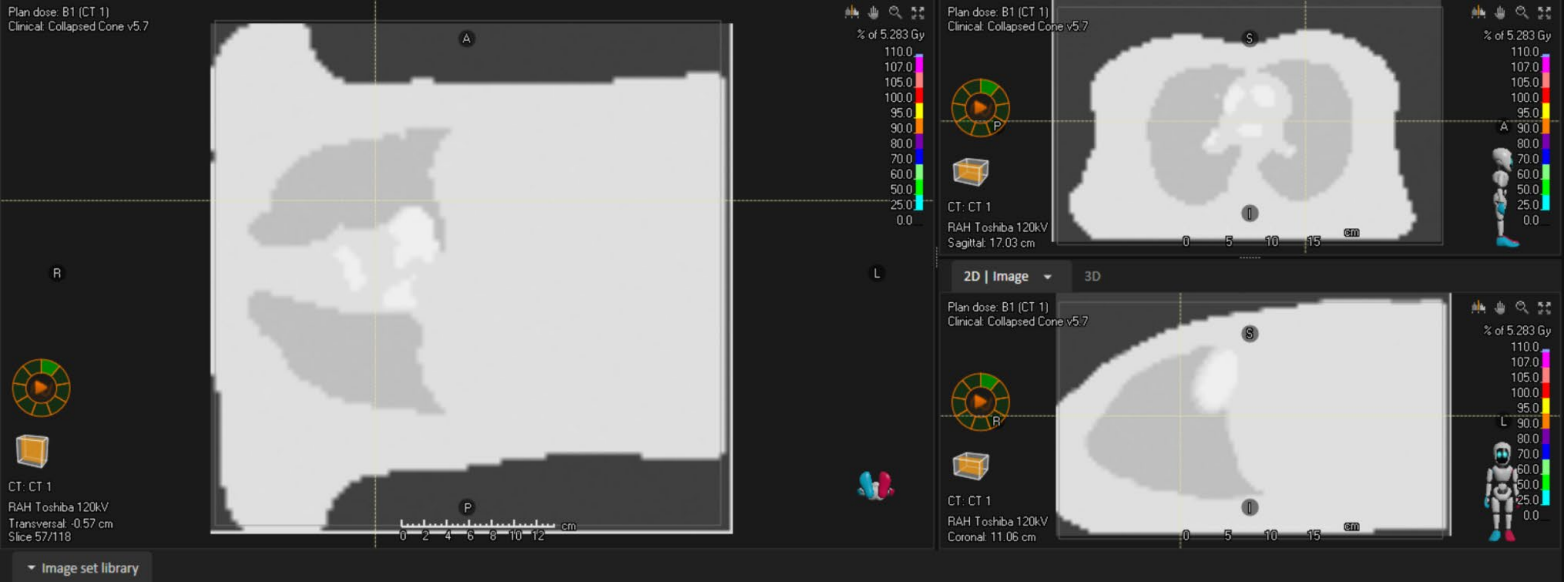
The 4D CT lung dataset in the Raystation treatment planning system
